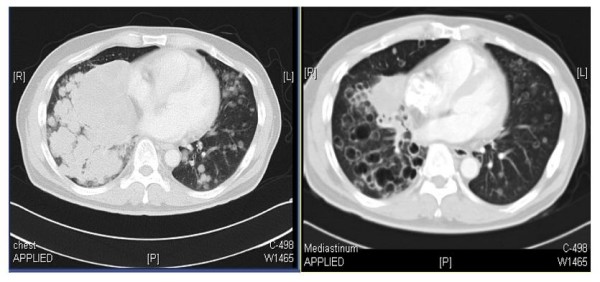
Computed tomography scan of tumor response and cavitation of lesions in a patient with metastatic lung carcinoma showing cavitation after 2 treatment periods. (with permission from Journal of Clinical Oncology) [64].
